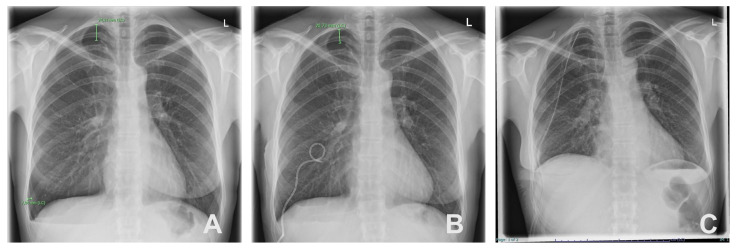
X-ray images of a female patient who suffered spontaneous pneumothorax for the first time at the age of 40. (A) Third recurrence at the age of 48. (B) Initial treatment using CT-guided drainage following pleurectomy. (C) Fully expanded lung following Re-VATS with talc pleurodesis.
